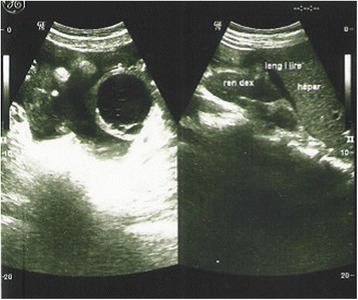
Abdominal ultrasound: cystic formation in left tubo-ovarial complex and ascites
